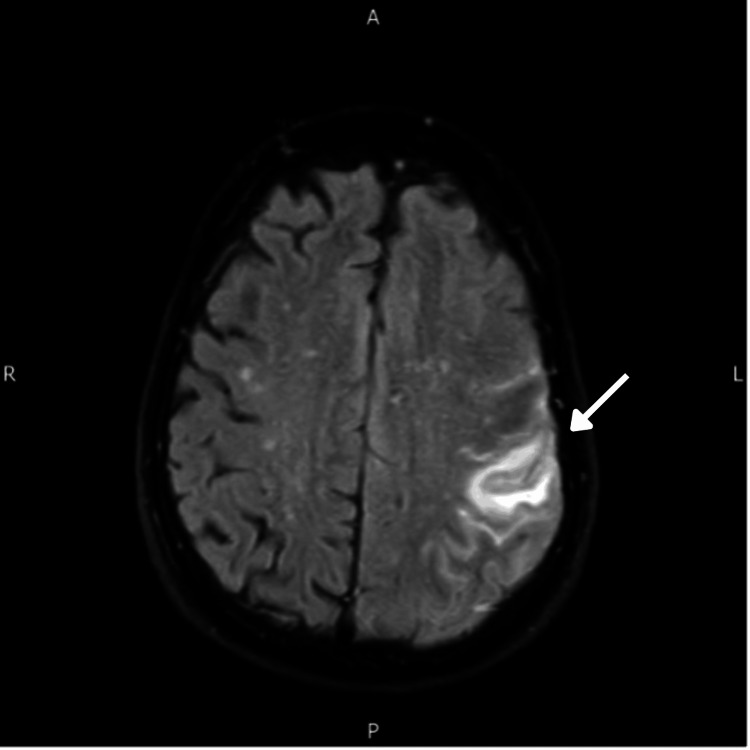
MRI April 2020 showing a new increase in T2 and FLAIR signal in the left temporoparietal lobe (arrow). MRI, magnetic resonance imaging; FLAIR, fluid-attenuated inversion recovery
